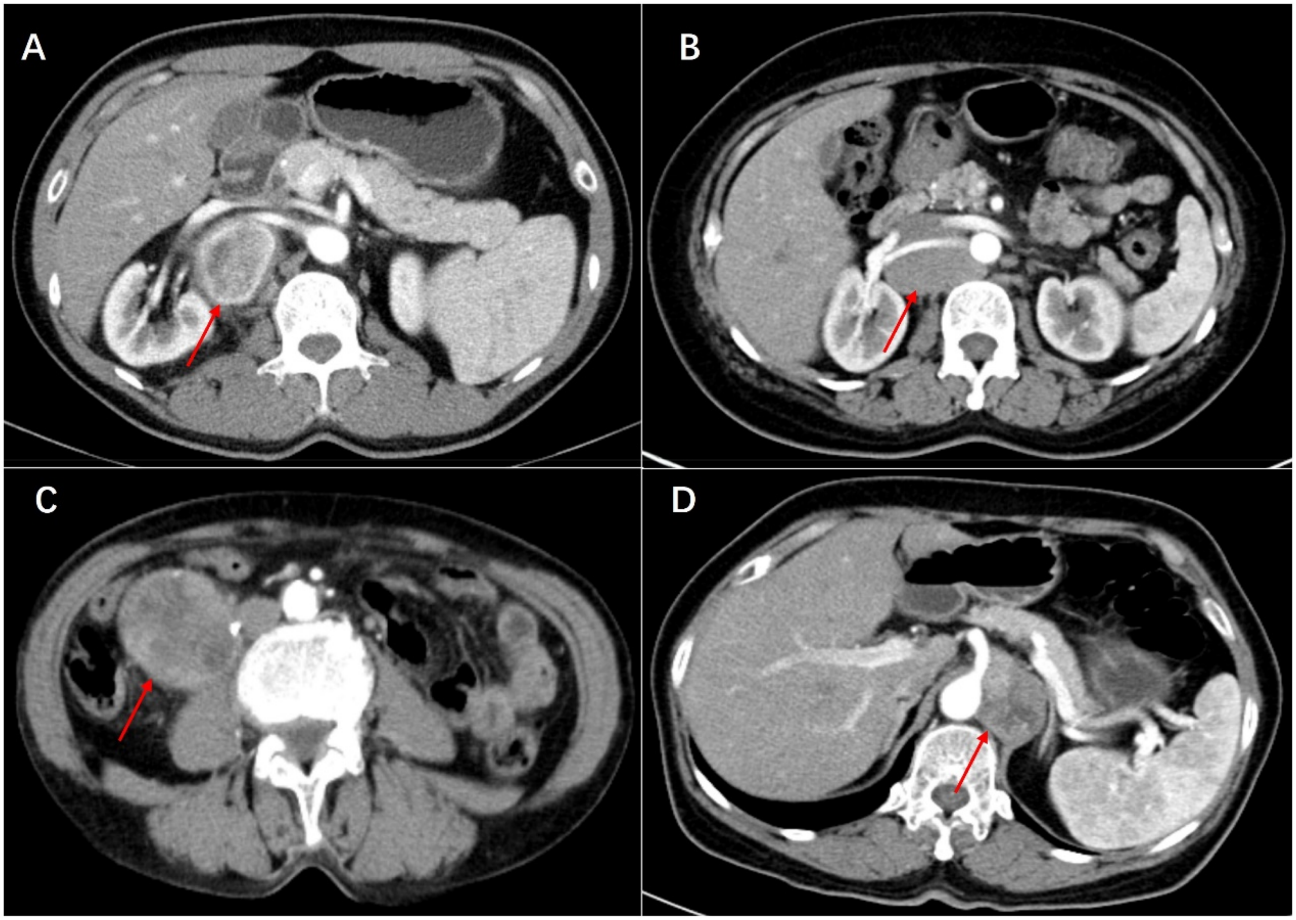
Artery phase of castleman disease (A), lymphoma (B), leiomyosarcoma (C) and paraganglioma (D) in enhanced CT scan. The tumors show heterogeneous enhancement in the arterial phase. All tumors are closely related to the blood vessels in the retroperitoneum, even encircling the renal artery or celiac trunk.
